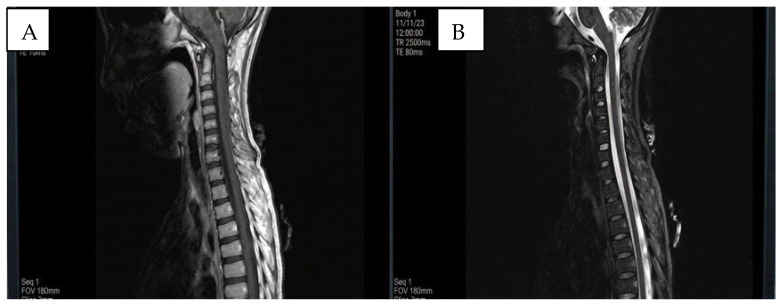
Non-contrast sagittal magnetic resonance imaging (MRI) of the cervicothoracic spine. (A) T1-weighted sequence demonstrating preserved vertebral bodies and no significant signal intensity alterations along the spinal cord. No obvious musculoskeletal abnormalities are noted. (B) T2-weighted sequence confirming the absence of pathological signal changes within the spinal cord path, with preserved surrounding musculoskeletal structures.
